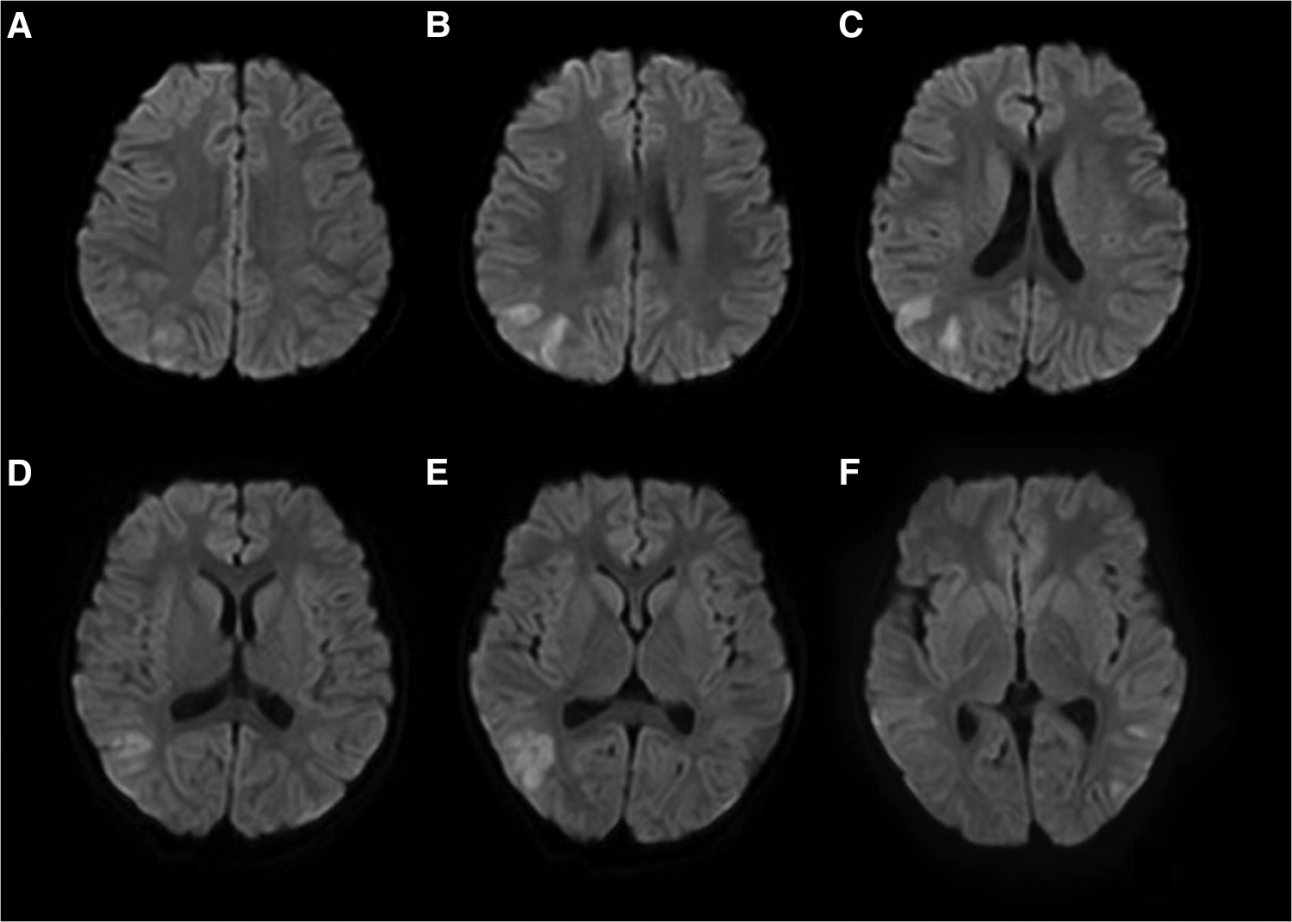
Brain MRI of patient #1 showed multiple high signal intensities on DWI in the right frontotemporal cortex (A–F).
